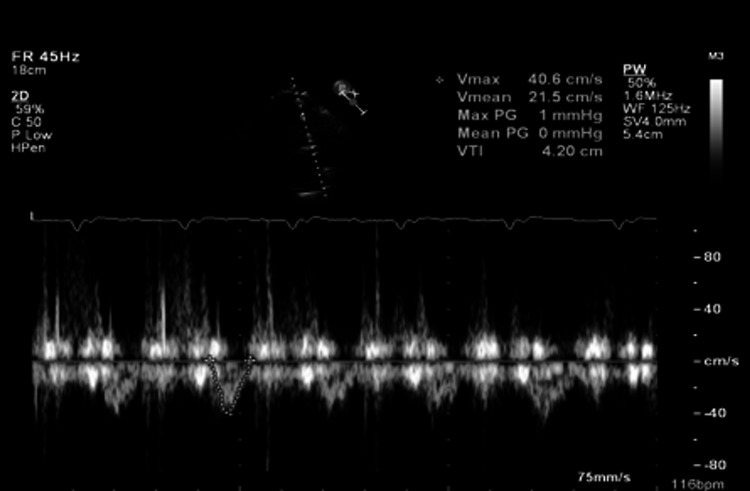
Doppler echocardiography showing a reduced right ventricular outflow tract velocity time integral of 4.20 cm
